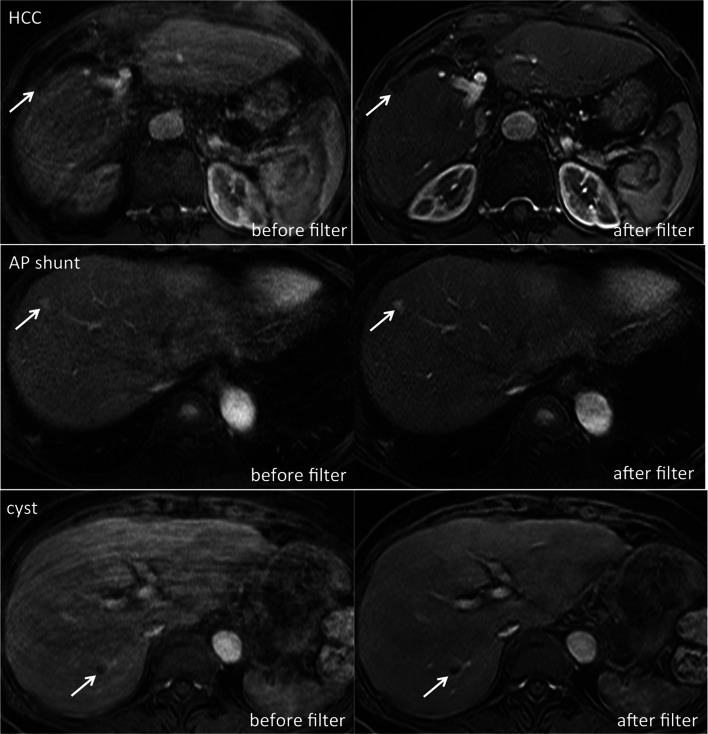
Lesion conspicuity before and after MARC application. Axial T1-weighted MR images in patients diagnosed with HCC, AP shunt and liver cyst (lesions are each indicated by arrow) show improved lesion conspicuity following filter application compared to the original images (conspicuity scores of 1, 2, and 1, respectively). Diagnosis of the liver cyst and AP shunt is greatly improved after MARC application, and identification of the HCC lesion, which could have easily be mist on the original images, is greatly improved
